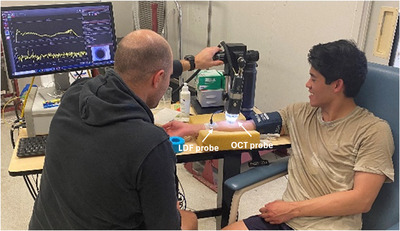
Instrumentation during the assessment of skin microvessels using OCT and LDF concurrently inside the controlled climate chamber following 50 min of either PH or HE. The OCT probe is attached to the skin adjacent to the LDF probe (<5 cm) and is affixed using double‐sided tape. Abbreviations: HE, heated exercise; LDF, laser Doppler flowmetry; OCT, optical coherence tomography; PH, passive whole‐body heating.
